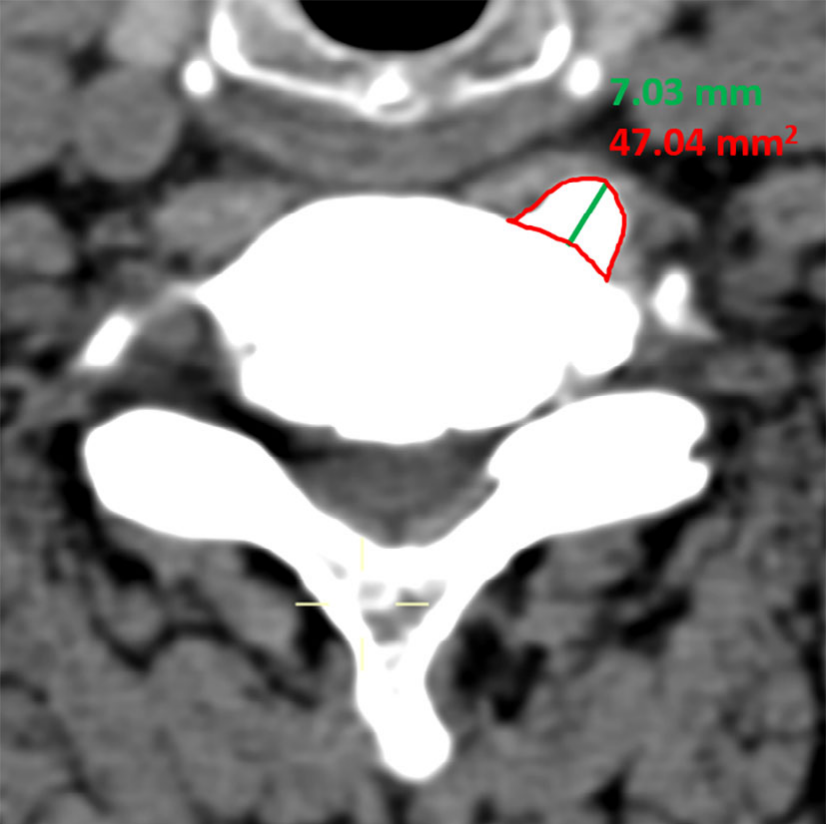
Radiographic measurements: Area of Luschka's joint osteophytes (red boundary), length of Luschka's joint osteophyte (green line).
